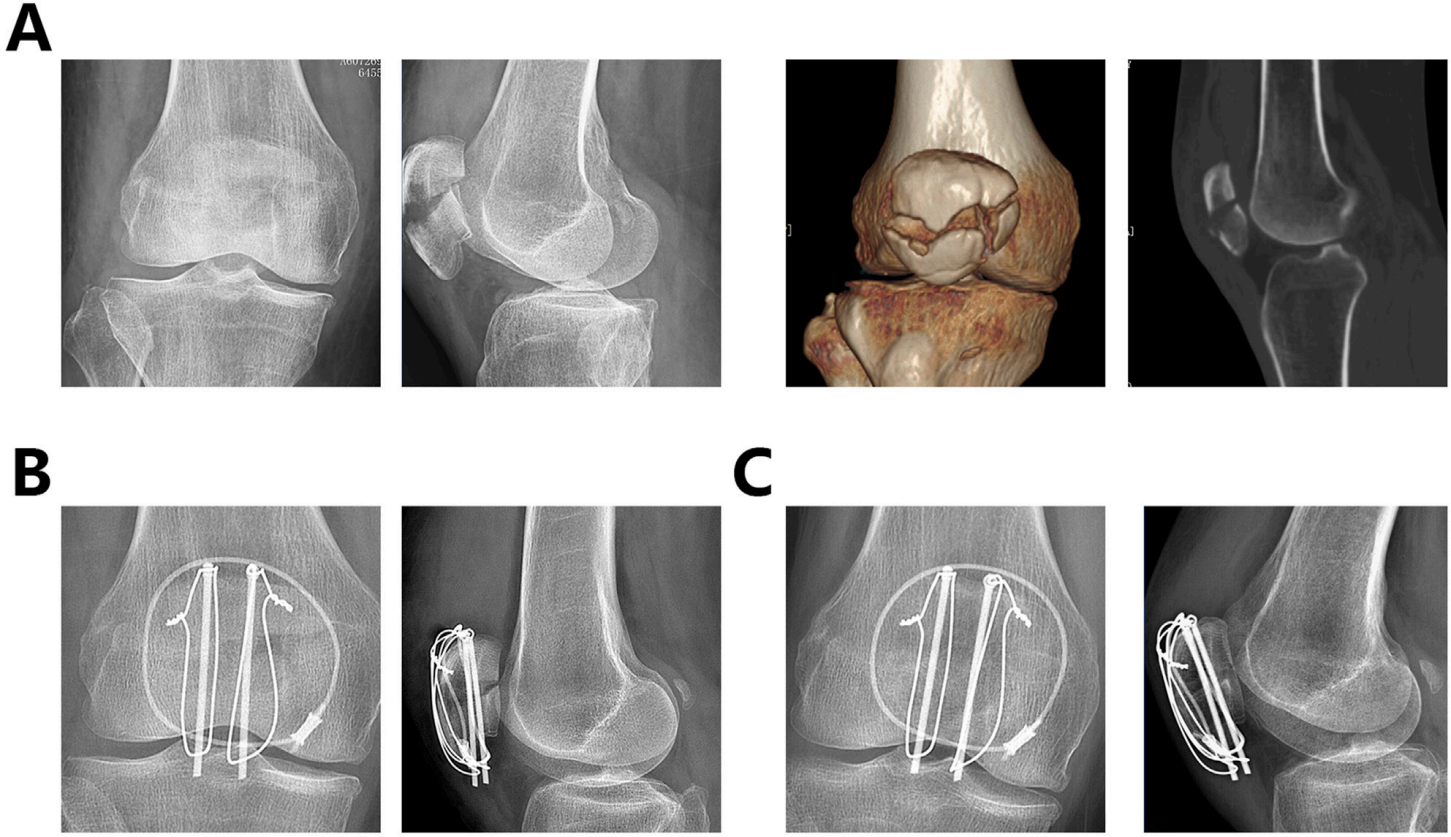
The radiographic images of a 67-year-old woman who underwent an internal fixation with the dual tension band technique. (A). Preoperative knee radiographs, CT scans, and 3D reconstructions (B). X-rays on the second postoperative day (C). X-ray 1 year after surgery.
